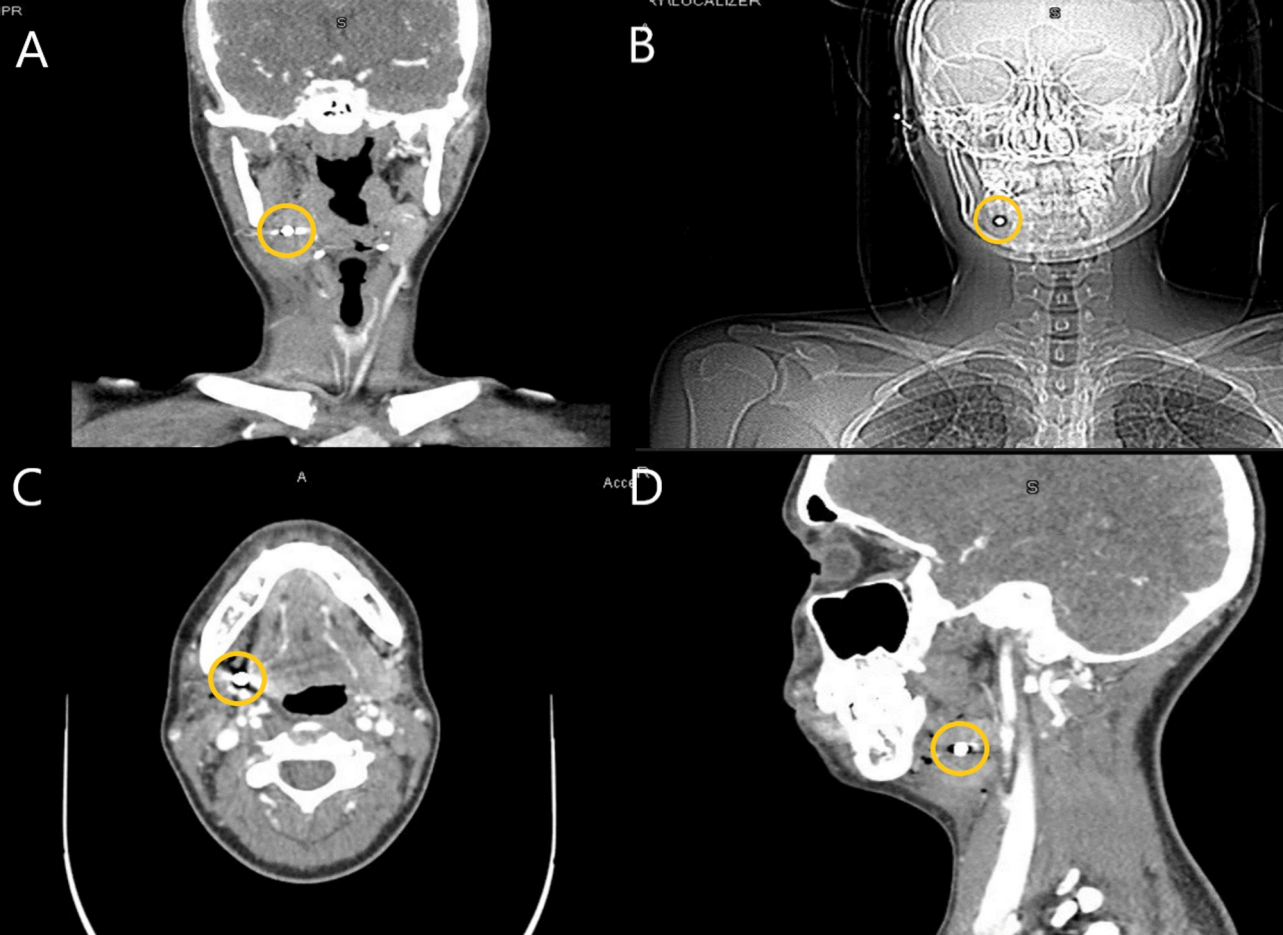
CT scan images from the coronal plane (A), localizer radiograph (B), axial plane (C), and sagittal plane (D) of the BB located in the submandibular gland BB, ball bearing
